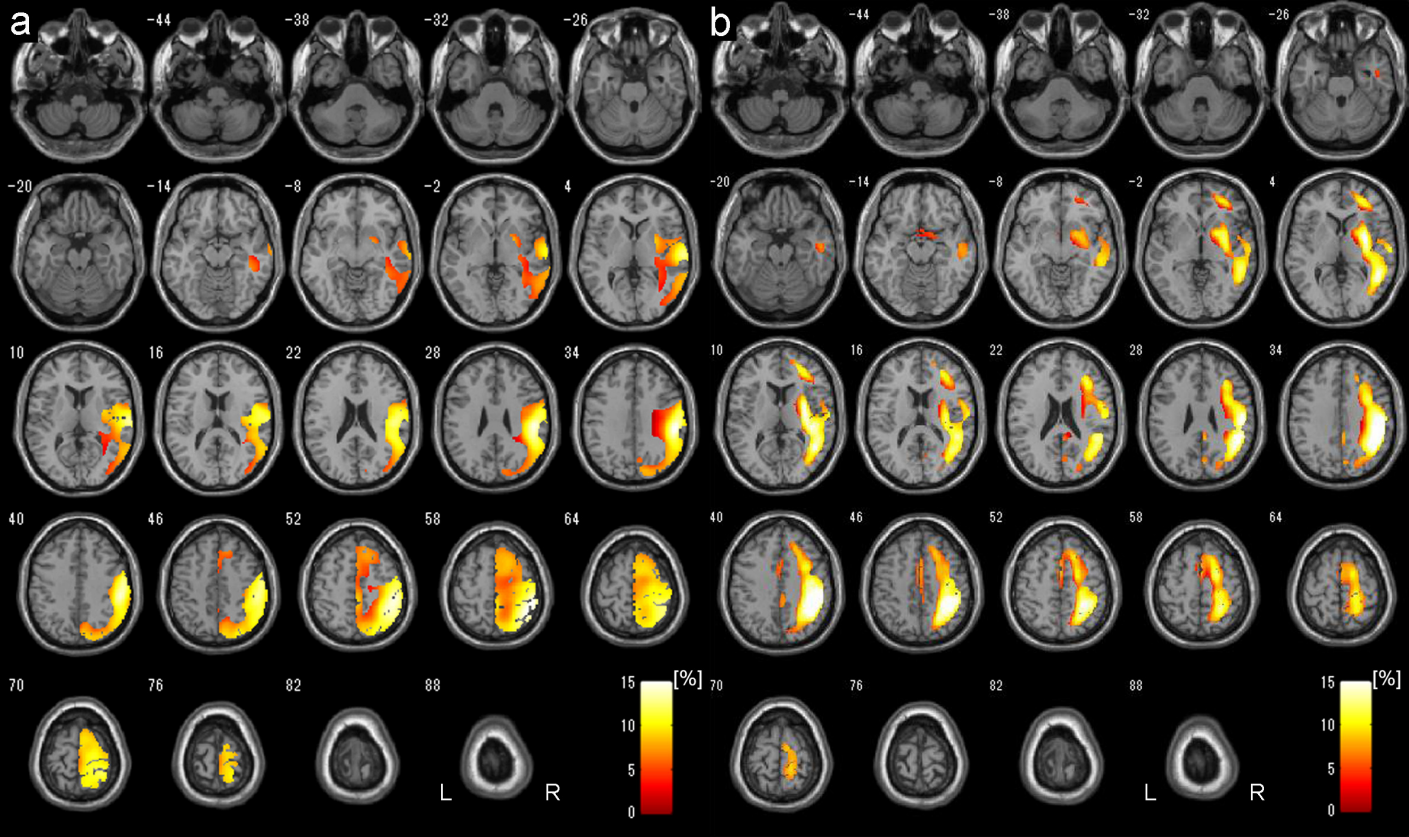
Postoperative CBF increased areas displayed in transverse sections. Increased ratio (%) at the area of significantly increased CBF on postoperative SPECT compared with preoperative SPECT is shown by using SPM8 with DARTEL (a) and SPM8 without DARTEL (a conventional method) (b). Images were reconstructed shown in 6mm thickness and the increased ratio was less than 15% in both methods. SPM8 with DARTEL revealed larger CBF increased areas (a), on the contrary, the conventional method showed relatively smaller CBF increased areas which were located in more medial regions compared with those with DARTEL.
